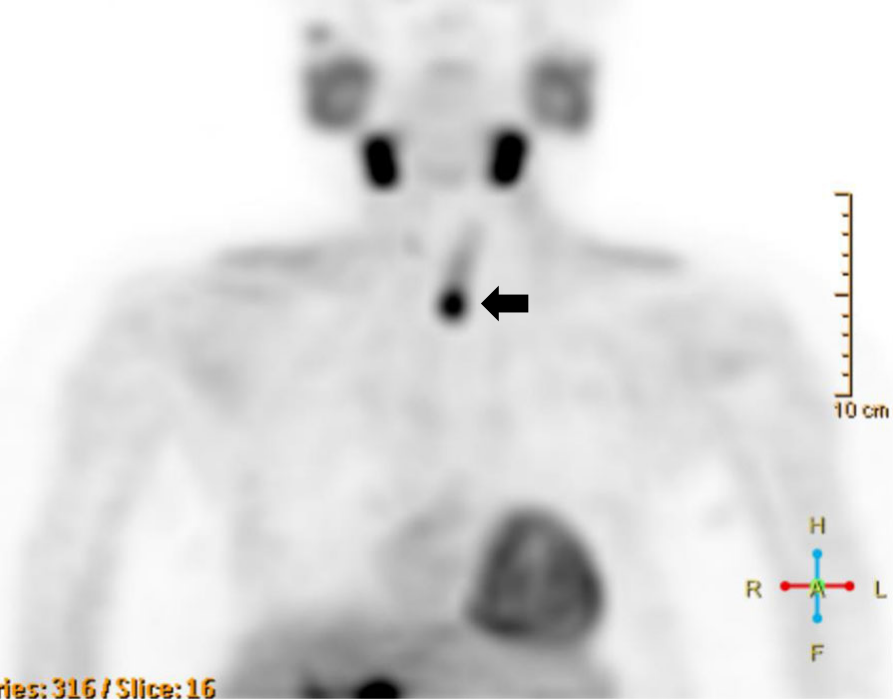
MIBI scintigraphy findings. MIBI accumulated in the lower mass (arrow) but not in the upper mass
